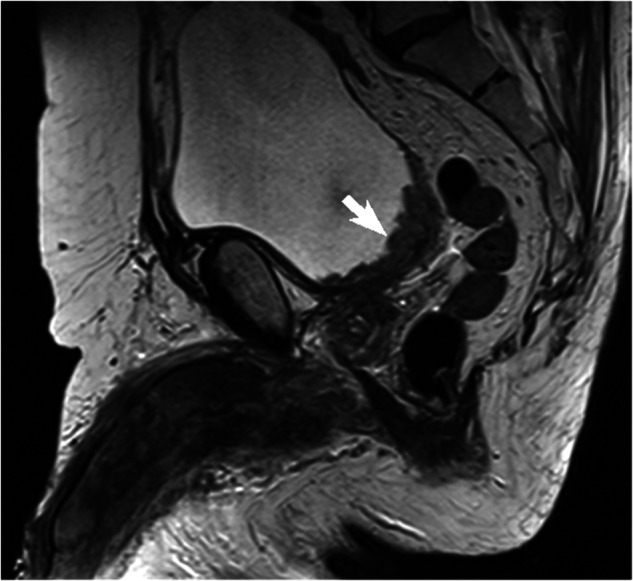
Sagittal plane T2w MRI pelvis showing a large plaque like bladder tumour (white arrow) that was confirmed transitional cell carcinoma. The abnormal low T2 tissue is seen extending through the bladder neck, prostate, and into the urethra and filling the penis with heterogenous low T2 tissue.
